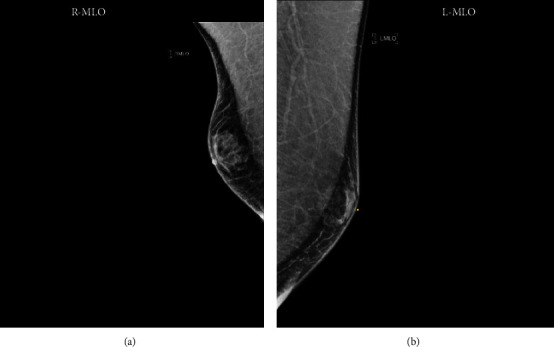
Magnetic resonance imaging (MRI) of the (a) right breast and (b) left breast showing flame-shaped subareolar densities consistent with gynecomastia.
